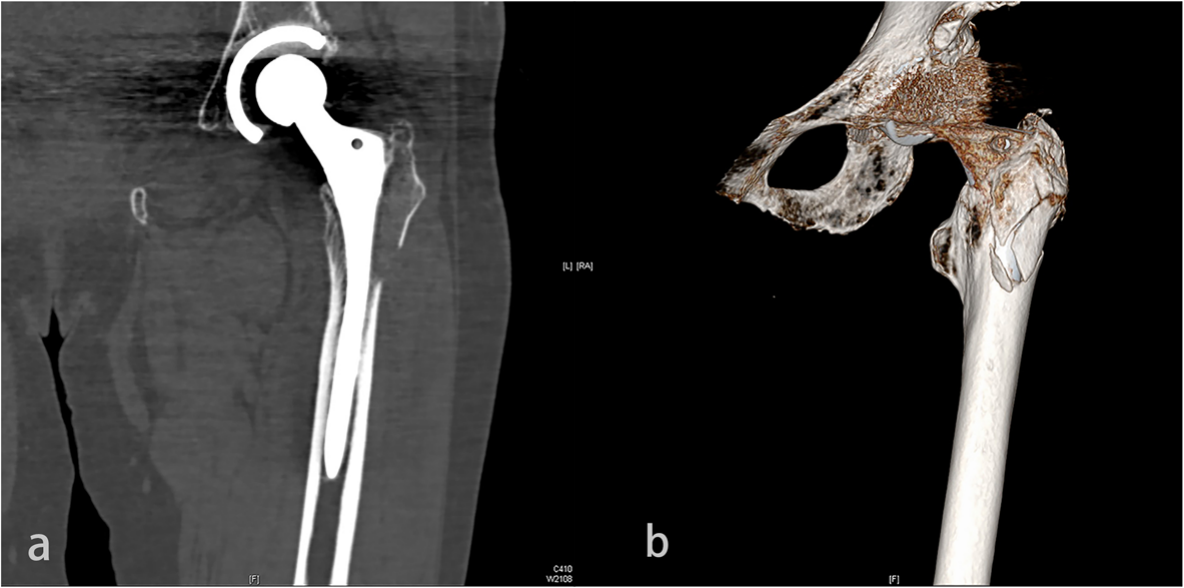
a-b Computed tomography scan and three-dimensional reconstruction of the left hip in postoperative day 1 showed the distal extension of the fracture line down the lateral cortex; this leads to destabilization of the stem because the lateral buttress is lost
